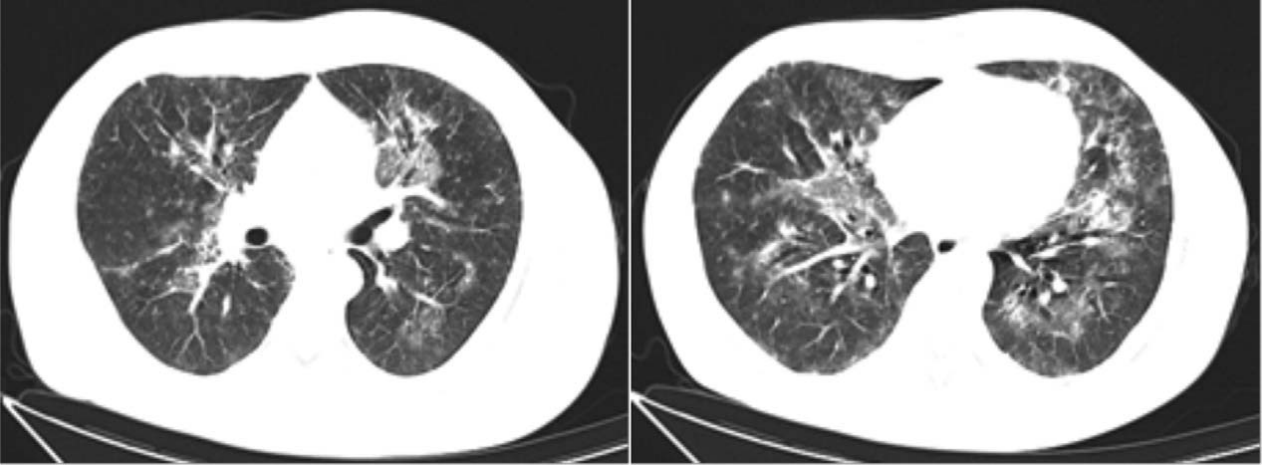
CT scan of the chest in outpatient department. CT scan showed exudative lesions in both lungs.
